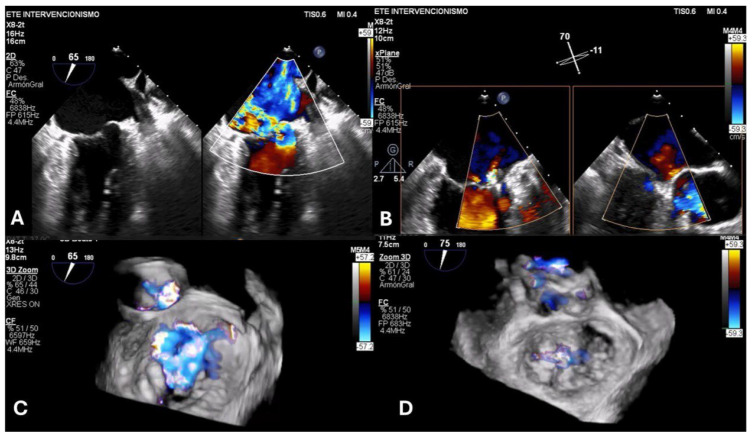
Redo M-TEER intervention. Transesophageal echocardiography interventional study (Courtesy of Li CP, MD). (A): Dual 2D and color Doppler imaging: Severe PMR before the index M-TEER procedure, secondary to P1 prolapse. (B): X-Plane color Doppler imaging: Optimal procedural result after M-TEER between A1 and P1. (C): 3D imaging: Severe recurrent MR one year after M−TEER. (D): 3D imaging: Final result after redo M-TEER with an additional device placed from A1−P1 to the nearby A2−P2 segment.
